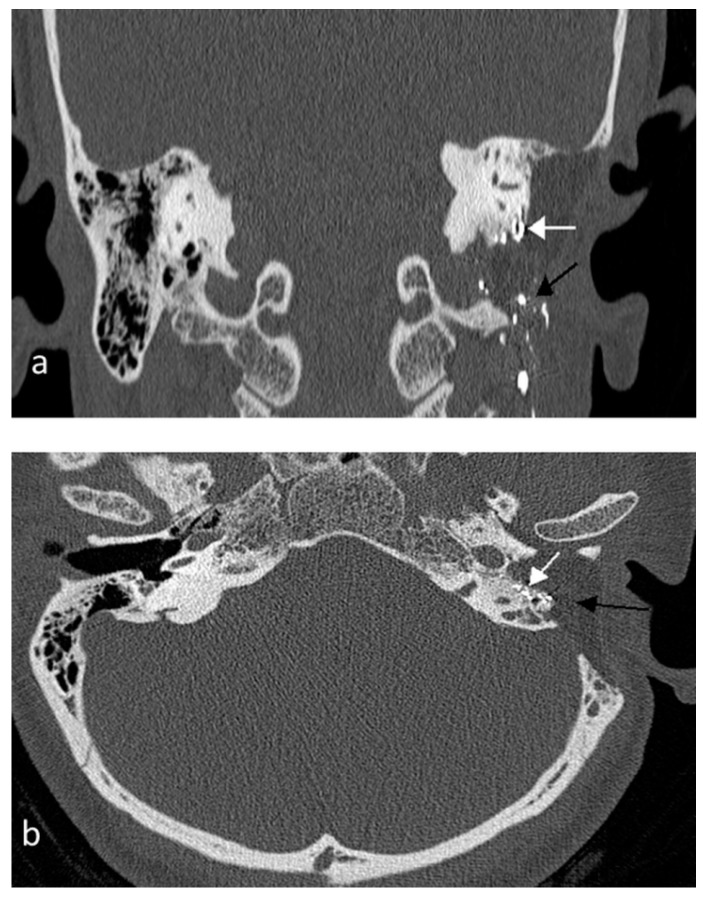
Post-operative computed tomography scan of temporal bone. (a) Coronal view shows hyper intense embolic material in the infralabrynthine (white arrow) and stylomastoid regions (black arrow). (b) Axial view shows middle ear and mastoid cavities filled with abdominal fat (black arrow); hyper intense embolic foci in the region of mastoid segment of the facial nerve (white arrow).
